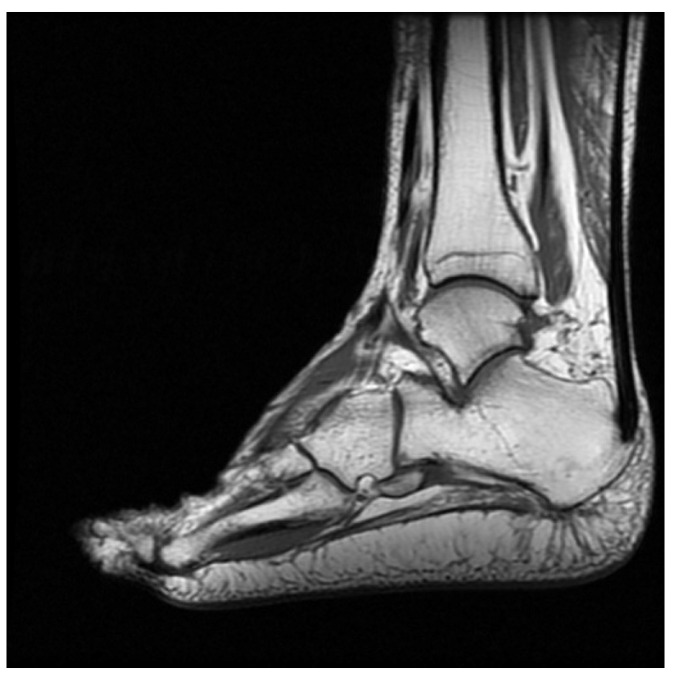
Postoperative MRI shows good tightening of the plantar fascia and the repair of the previous lesion.
